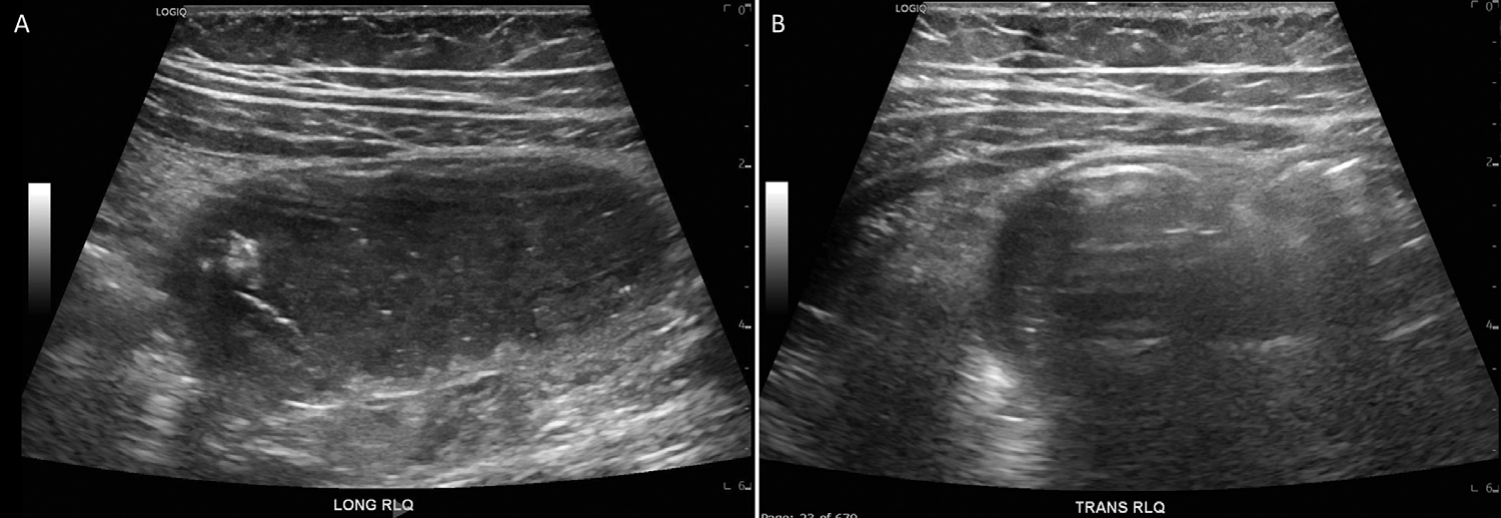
Focused ultrasound examination of the abdomen was performed. A hypoechoic focus was noted in the right lower quadrant, sagittal plane (Fig. 2A) and transverse plane (Fig. 2B). This lesion demonstrated posterior acoustic shadowing. Of note: Appendix was not visualized, perhaps related to projections for surrounding bowel loops.
